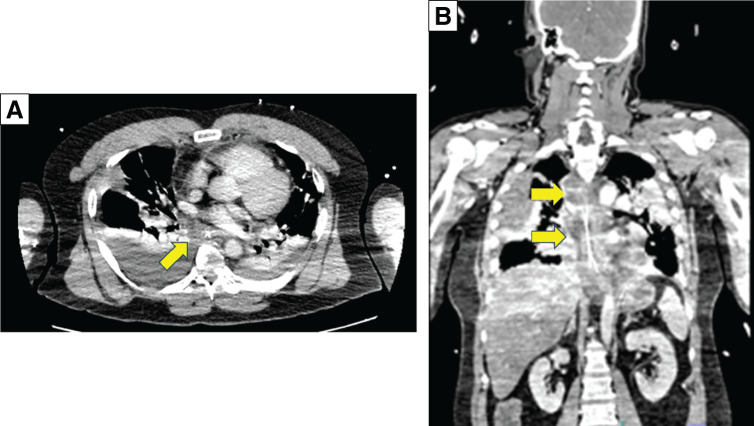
CT images of the chest demonstrating mediastinitis. (A) Axial contrast-enhanced chest CT shows increased fat density extending from the upper to the posterior mediastinum (yellow arrow). Bilateral pleural effusions are present, with loculated fluid collection on the right side. (B) Coronal contrast-enhanced chest CT confirming the extent of mediastinal inflammation and the presence of bilateral pleural effusions (yellow arrows).
